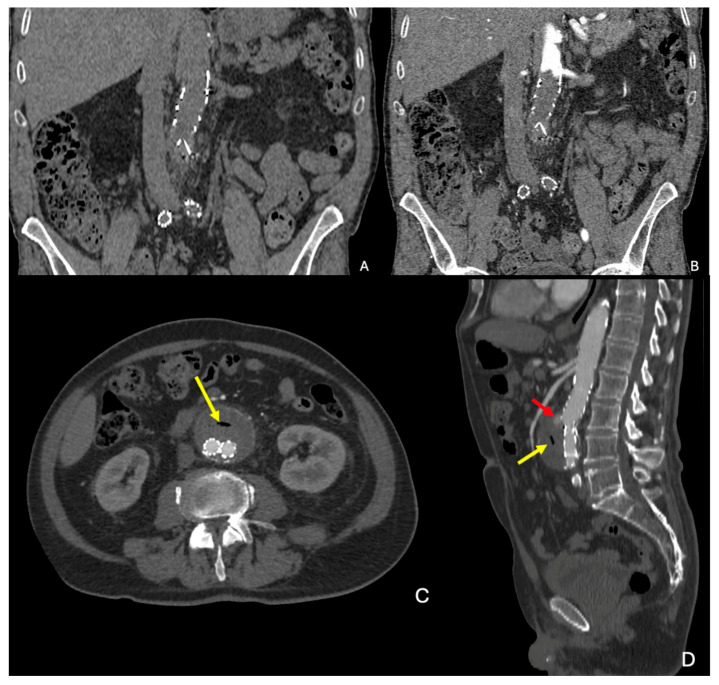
(A,B) Unenhanced and enhanced CTA showing a coronal view of a stent graft occlusion of a patient admitted to the emergency room for abdominal pain, who had undergone EVAR 4 years before. (C,D) Axial and sagittal enhanced CTA of another patient showing an infected EVAR graft with air bubbles (yellow arrow), mild fat stranding, and type 2 endoleak coming from the IMA (red arrow). IMA: inferior mesenteric artery.
